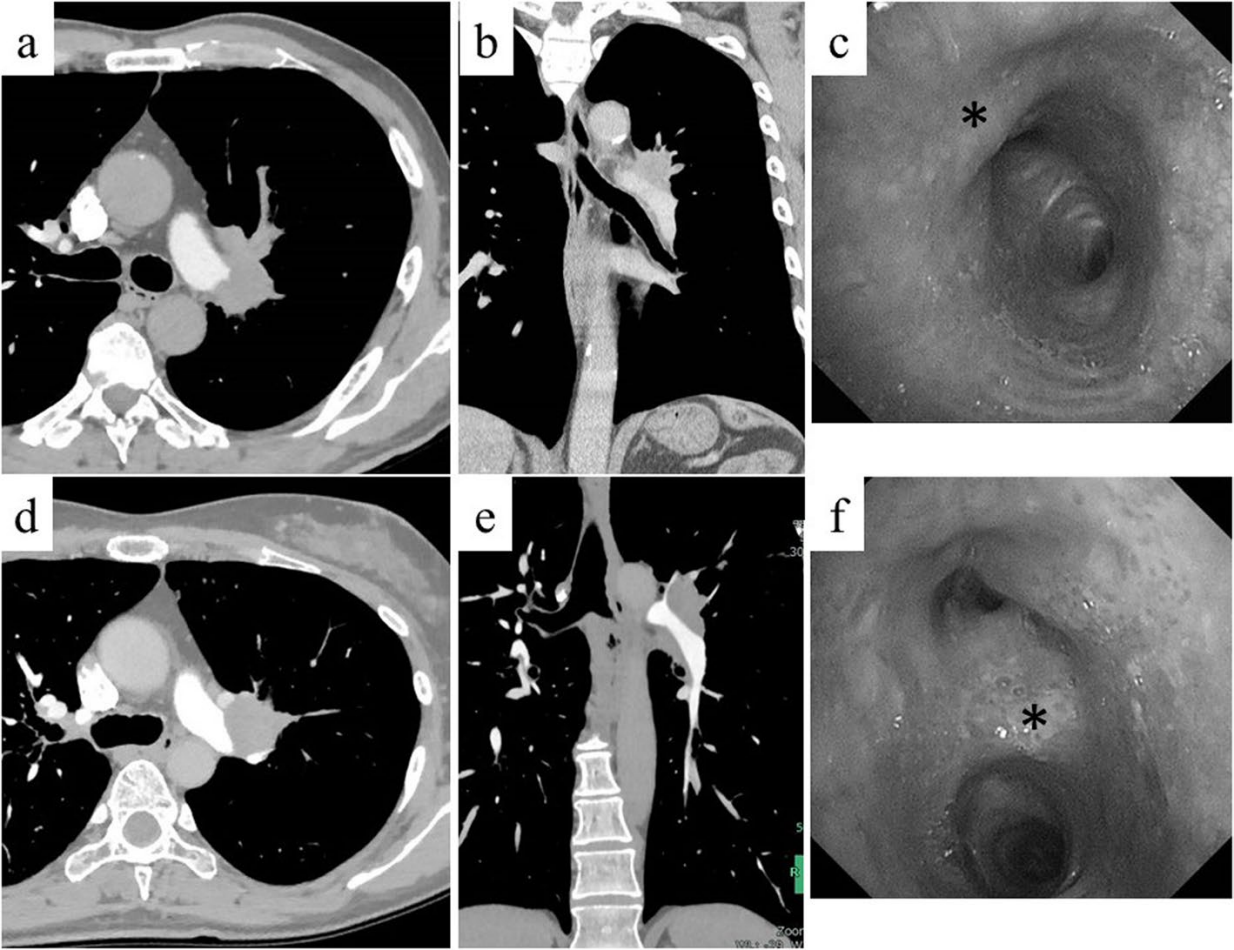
Chest computed tomography showing the pulmonary hilar tumor in the left upper lobe. The tumor was suspected to have invaded the long segment of the PA (a, b case 1; d, e case 2). Bronchoscopy showed the exposition of the tumor to the left upper bronchus. The tumor was diagnosed as squamous cell carcinoma by TBB from * site (c case 1—orifice of left upper bronchus; f case 2—second carina)
